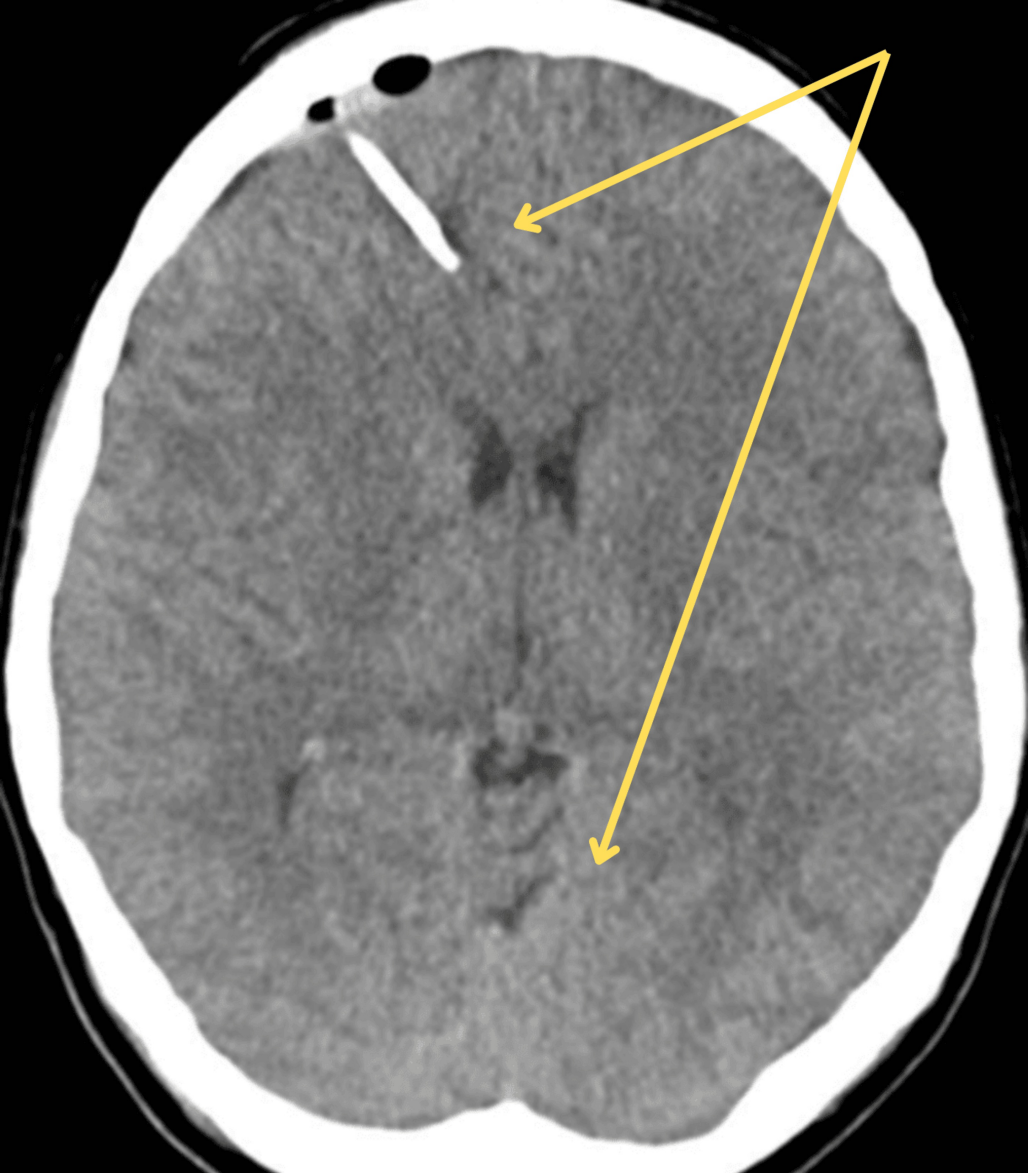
Follow‑up non‑contrast axial computed tomography (CT) of the brain after 24 hours of therapy showing reduction of cerebral edema with the restoration of ventricular and sulcal anatomy compared to the admission scan
